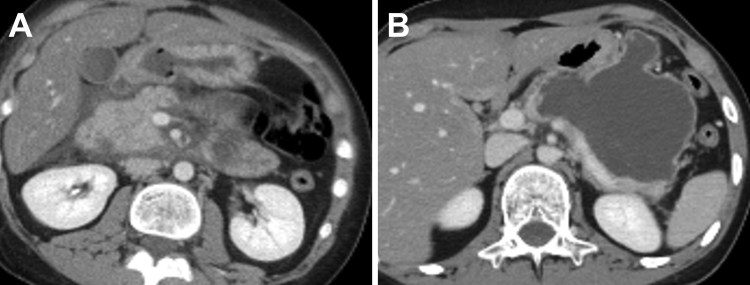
Evolution from acute necrotizing pancreatitis to walled-off necrosis (WON). (A) Contrast-enhanced CT at the onset of acute necrotizing pancreatitis. (B) Follow-up CT demonstrating the development of a large walled-off necrosis. CT, computed tomography.
